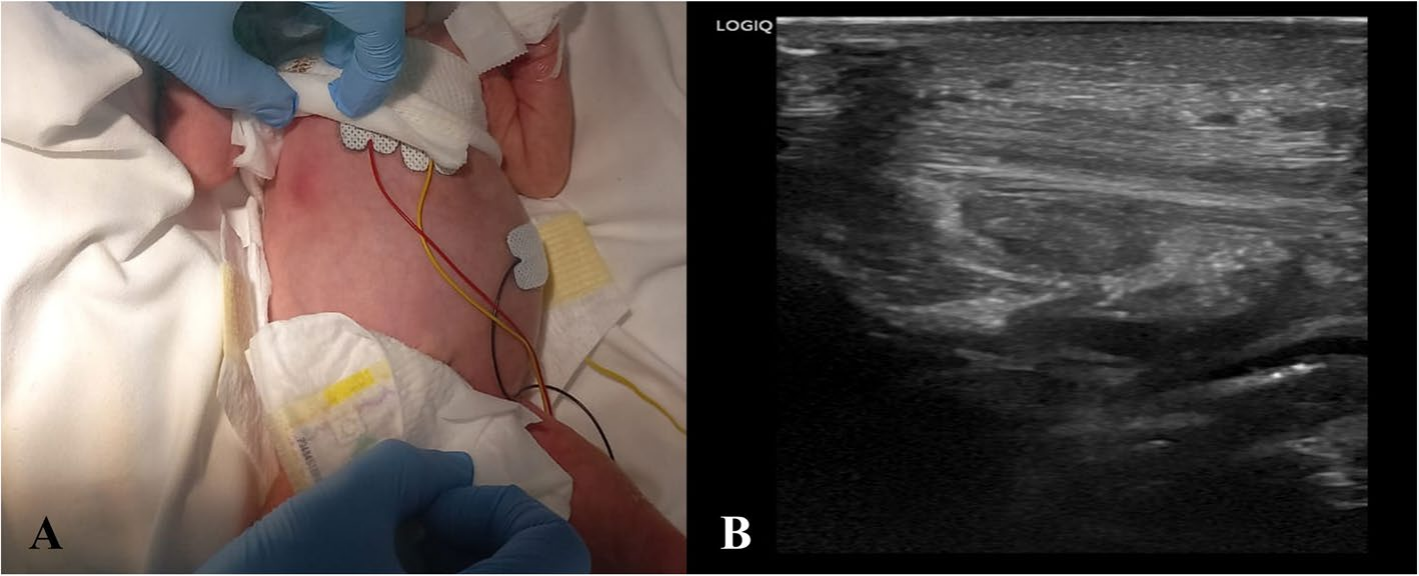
Case #1 clinical observation of a hyperaemic, painful, swelling of taut and mobile consistency mass appeared on the thorax of the baby, in correspondence to the twelfth right rib (A). Ultrasound scan of the mass which shows an oval image with a major axis of about 1.3 cm, with a hypoechoic eco-structure, finely corpuscular (B)
